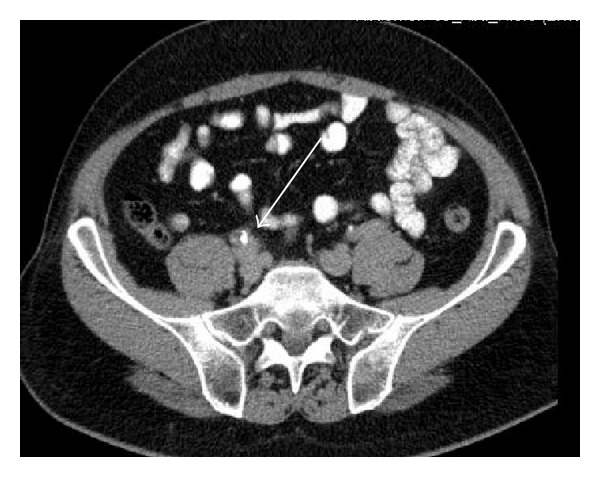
Thin-layer computed tomography demonstrating a small thickening of the right mid ureter.
